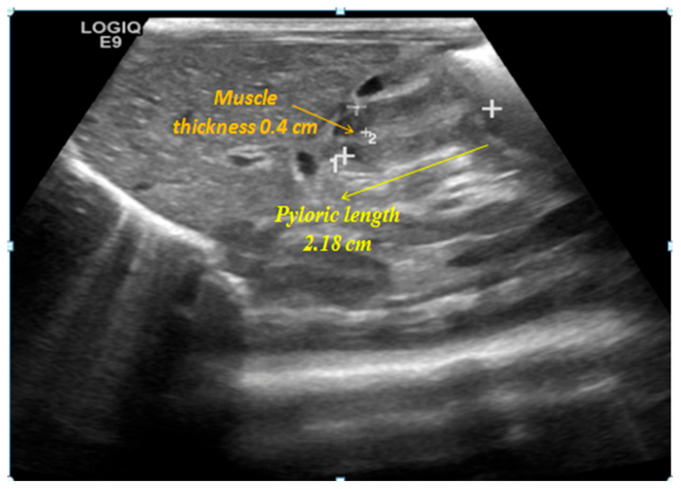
Abdominal ultrasonography demonstrating hypertrophic pyloric stenosis.
